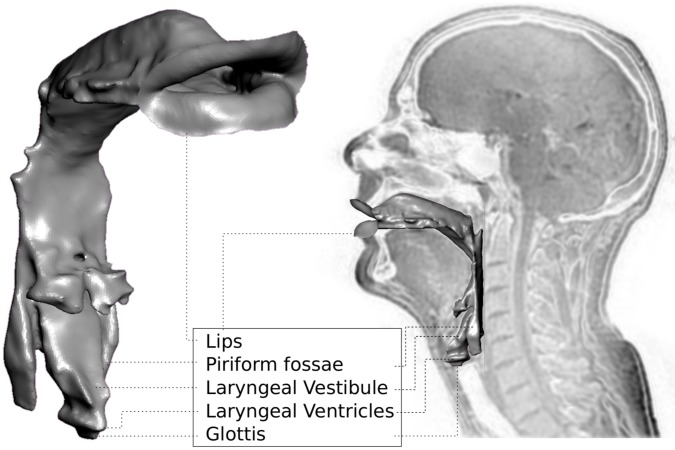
The hypopharynx cavities in the Vocal Tract. Vocal Tract profile superimposed on an MRI midsagittal slice of a singer while phonating on the vowel as in the word /st
